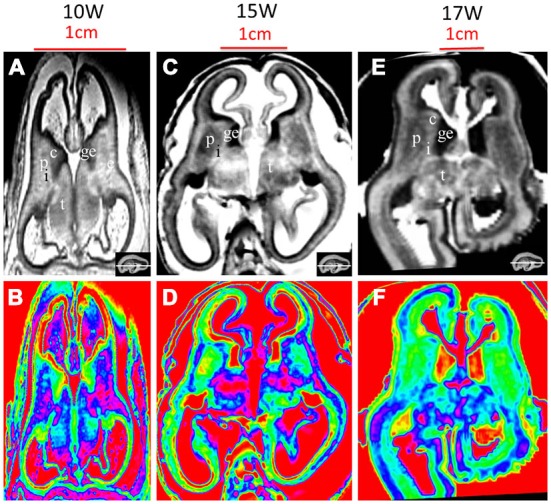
T2-weighted axial MR grayscale images and color images at 10 GW (A,B), 15 GW (C,D) and 17 GW (E,F). During develoment, the cerebral walls became thicker but with heterogeneous speed; the laminar structure became clearer and the proportion of ventricles got smaller. Nucleus-rich structures such as the thalamus, putamen and caudate nucleus became enlarged and their signals were low and heterogeneous on T2-weighted grayscale images; the signal of the internal capsule and external capsule became lower, and the ganglionic eminence became enlarged. See text for details. c, caudate nucleus; ge, ganglionic eminence; p, putamen; i, internal capsule; e, external capsule; t, thalamus. The white line in each panel shows the location of the corresponding coronal slice.
